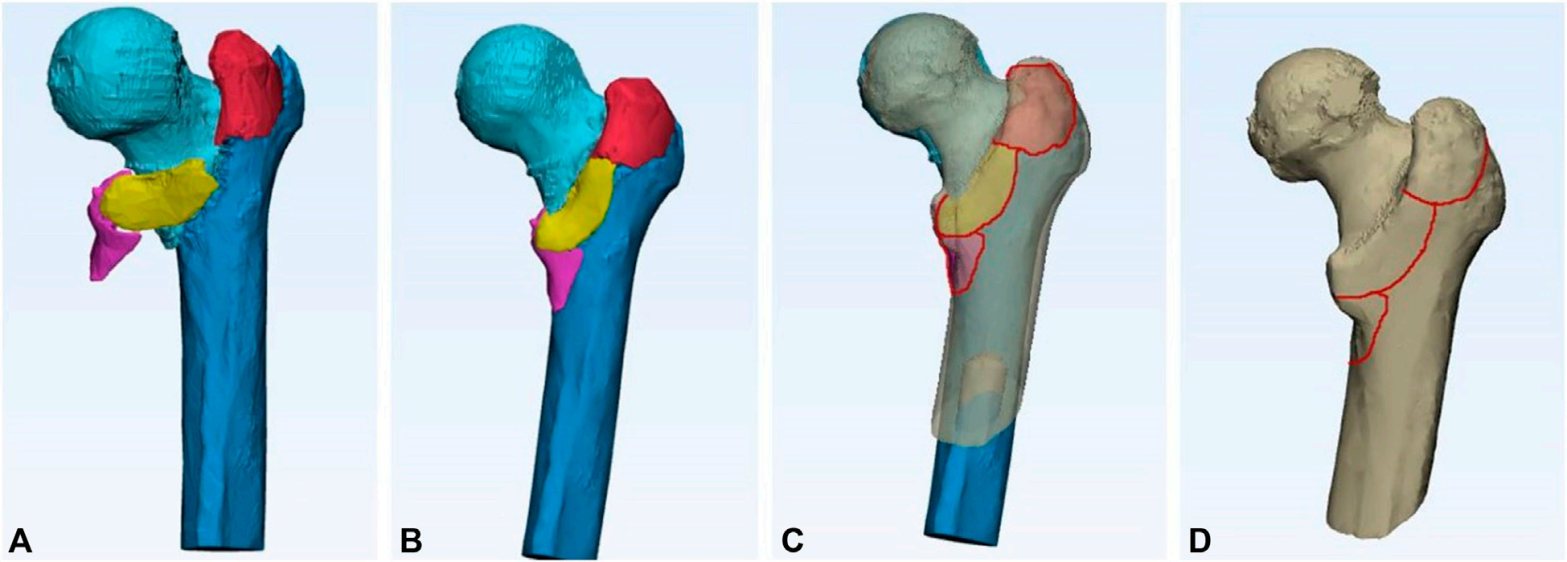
Three-dimensional fracture-mapping technique is used to prove the spatial morphology of femoral intertrochanteric fracture. CT data were used for reconstruction and virtually reduced fractures (A,B). Then, if necessary, other processes were performed to rotate, normalize, and flip the image to best match the 3-dimensional template of the femoral trochanter (C). Smooth curves are directly drawn on the surface of the 3-D model to represent fracture lines (D).
